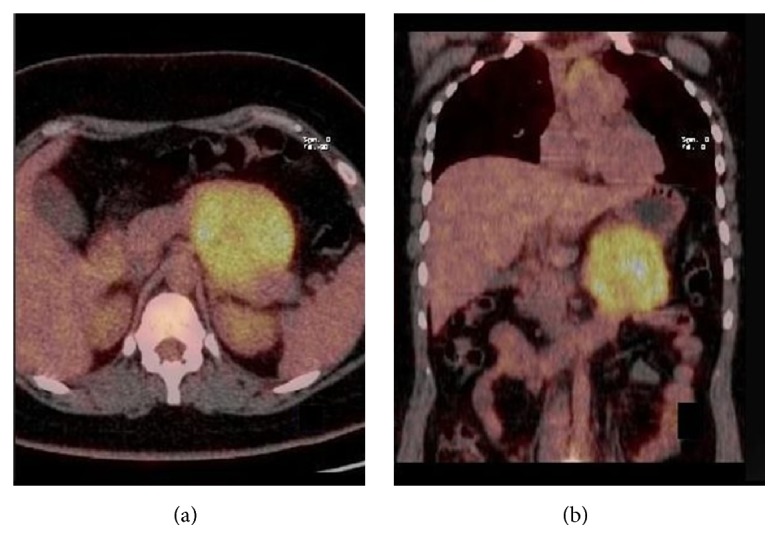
Positron emission tomography with CT acquisition (PET/CT) of the abdomen: axial (a) and coronal image (b) showing a pathologic uptake of FDG in a well-circumscribed, round mass in the tail of the pancreas (case number 15).
